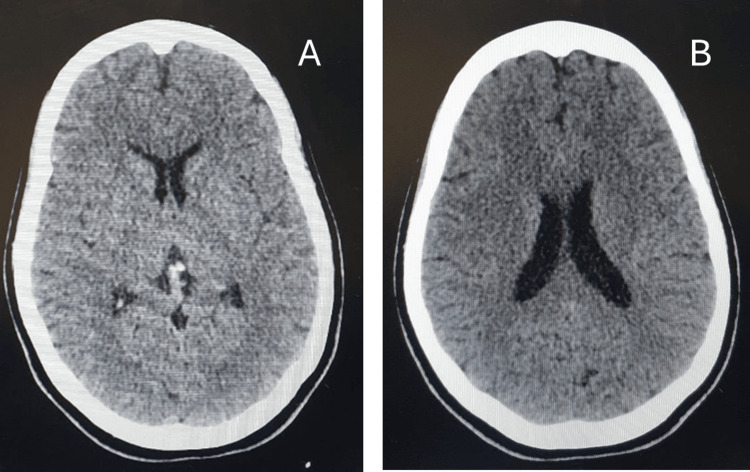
Cranial computed tomography (CT) (A) Non-contrast computed tomography (CT) of the brain showing an inferior axial section. No acute intracranial pathology or significant abnormalities were identified. (B) Non-contrast computed tomography (CT) of the brain demonstrating a superior axial section. No evidence of acute intracranial pathology or clinically relevant findings was identified
